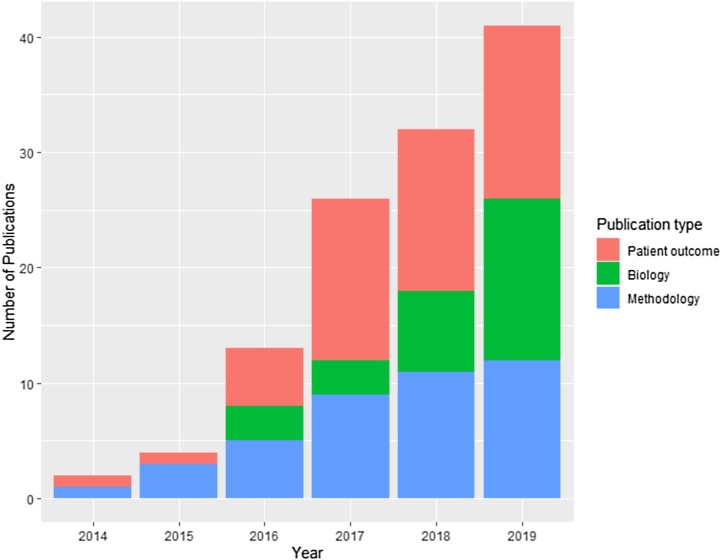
Frequency of CT NSCLC radiomics studies published from 2014 to 2019. Publications are categorized as those investigating radiomics methodological concerns, those evaluating radiomic signatures as prognostic or predictive biomarkers of patient outcome, and those evaluating radiomic signatures as biomarkers of tumor biology.
